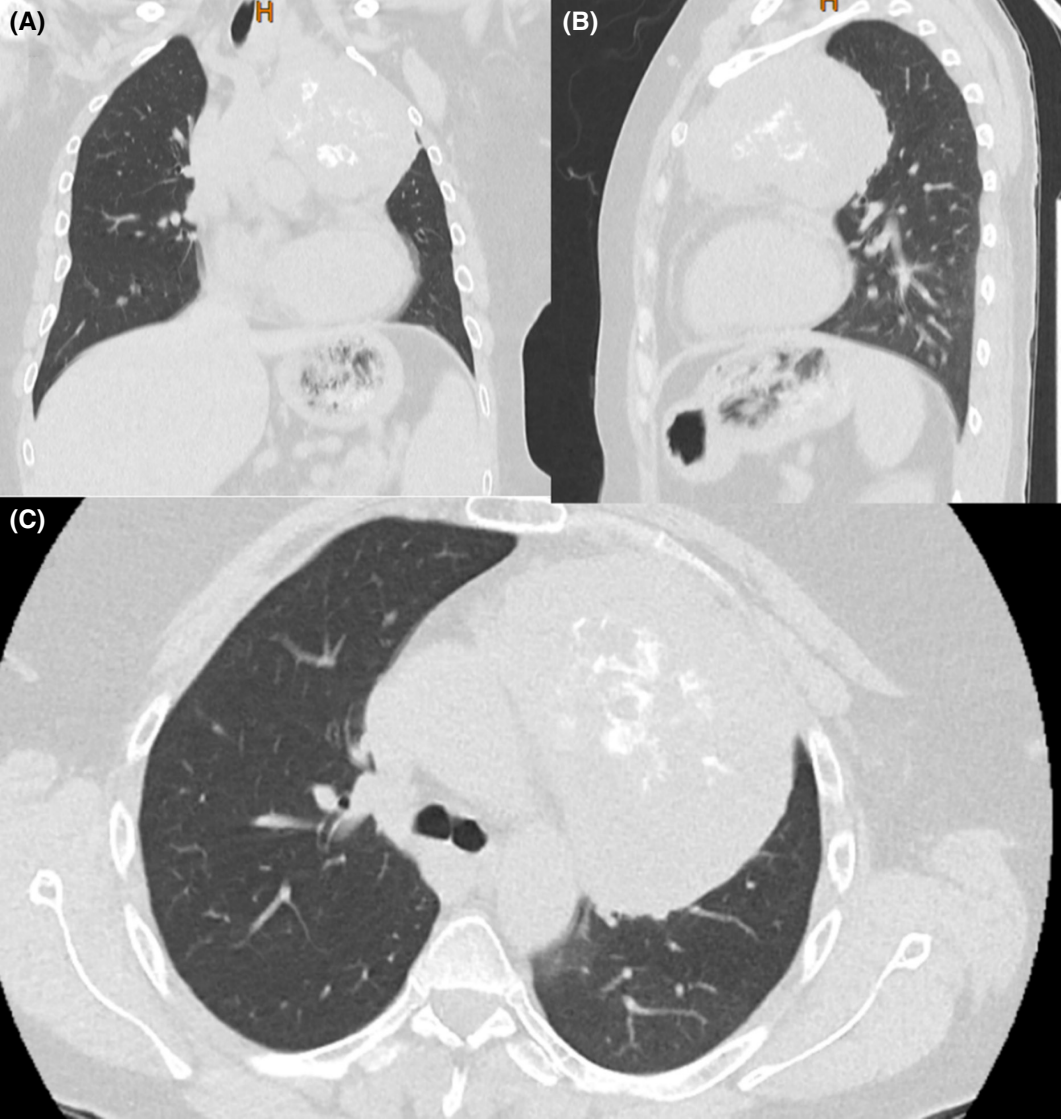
Chest computed tomography scan of a 42‐year‐old patient with a mediastinal thymoma; (A) coronal view, (B) sagittal view, and (C) axial view
